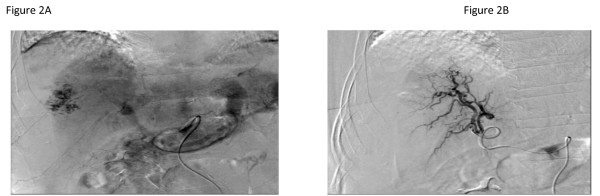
The celiac trunk angiogram showed a hypervascular mass in right lobe of liver with contrast extravastion from the tumor surface (black arrow), which was compatible with CT findings of a hypervascular hepatic tumor bleeding. Figure 2B-After embolization, no contrast extravastion from the tumor was shown.
